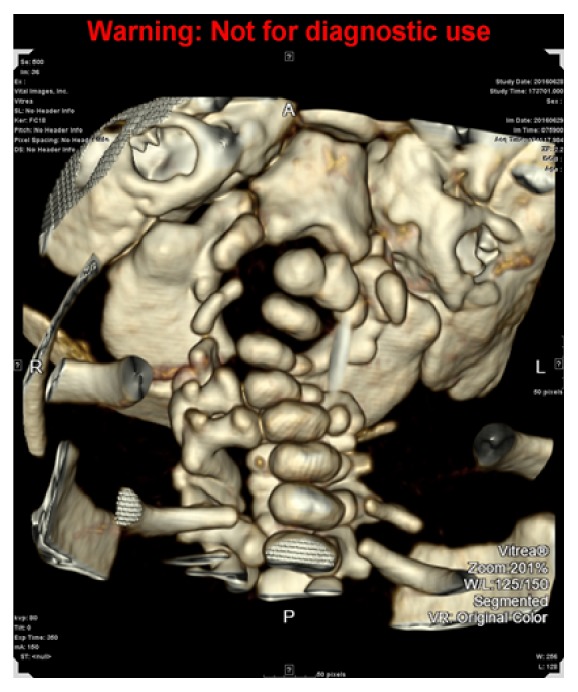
3D image of MRI of cervical spine: absent pedicle in the midcervical spine.
